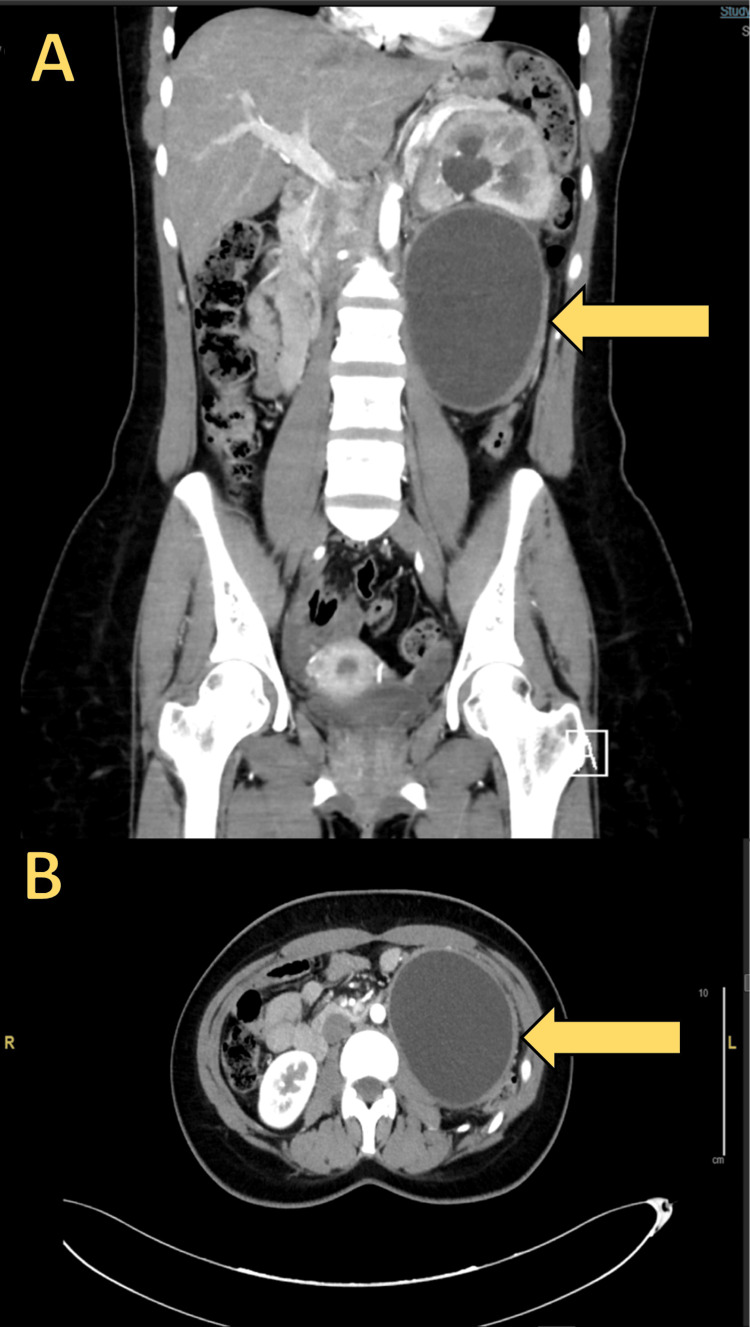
The CT scan shows a large, thick-walled cystic retroperitoneal mass containing a small amount of fat at the superior portion. (A) coronal plane of the abdominal CT; (b) transverse plane of the abdominal CT
